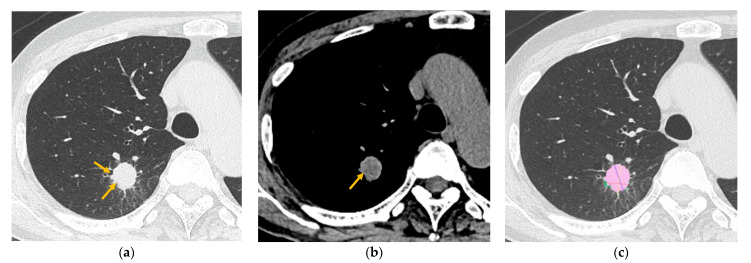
A 70-year-old man with invasive adenocarcinoma (positive for STAS). (a) High-resolution computed tomography (HRCT) revealed a solid nodule with lobulation (arrows). The diameters of both the tumor and solid components were 21.0 mm. The rate of the solid component diameter was 100%. (b) Central low attenuation was observed (arrow). (c) The volume of the tumor and solid component were 2954.7 mm^3^ (pink and green area) and 2954.3 mm^3^ (pink area), respectively. The rate of the solid component volume was 99.986%. Note—STAS, spread through air spaces; HRCT, high-resolution CT.
